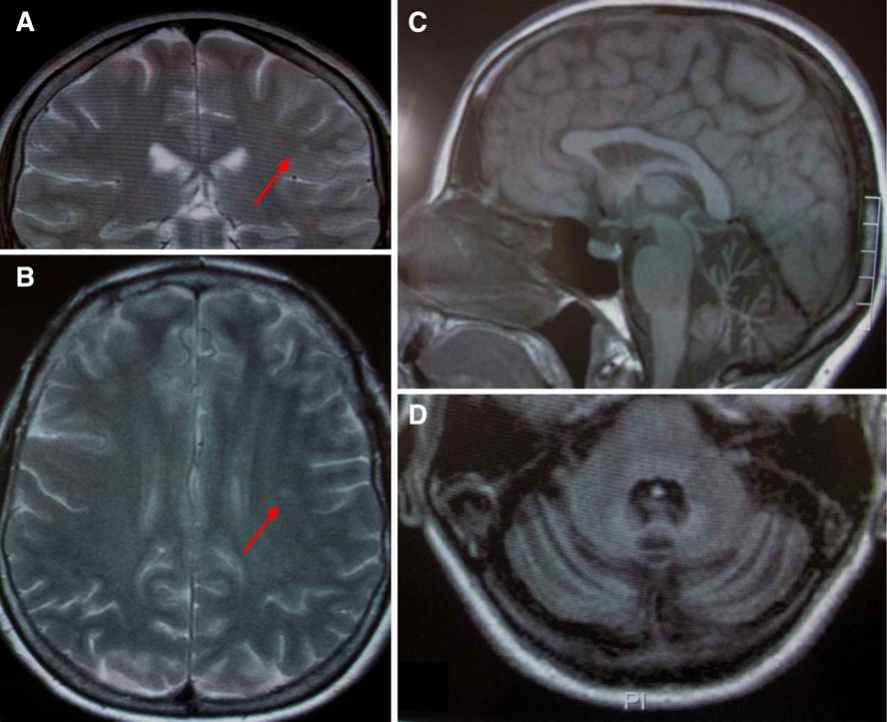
a, b Coronal and axial T2-weighted images showing the small vascular lesion near the left silvian scissure (*red arrow*). c, d Axial and sagittal T1 weighted images demonstrating cerebellar atrophy with deeper sulci on (color figure online)
